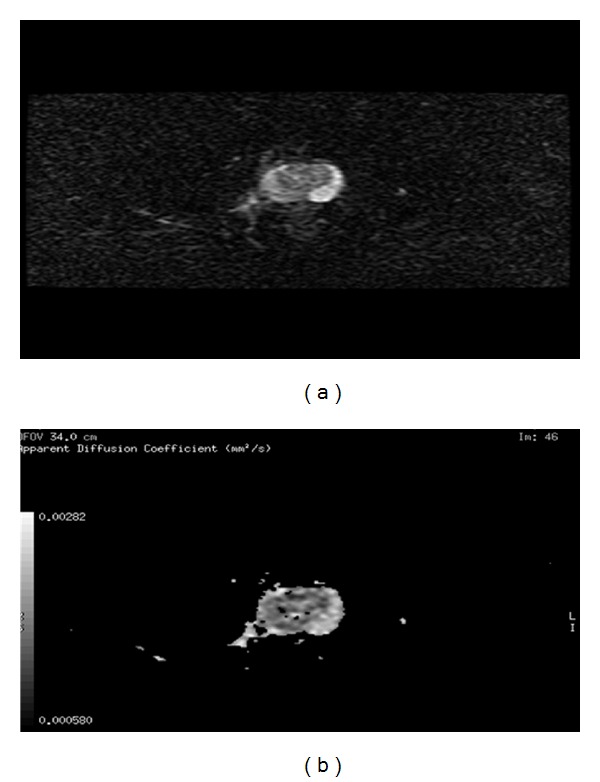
Example of 62-years-old male patient with cancer prostate and PSA >10 ng/mL. At the corresponding DWI/ADC map (a) there is a relative hyperintense lesion at the medial aspect of the right PZ at *b* = 800 and it is hypointense at *b* = 0 and at ADC map (b).
